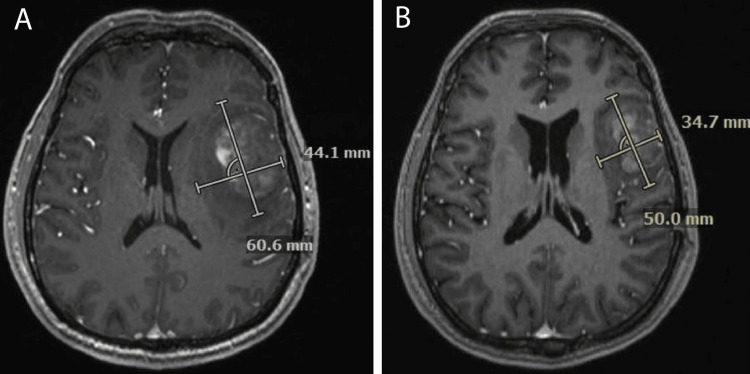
Radiographic response after three cycles of azacitidine and venetoclax Axial MRI brain with contrast. A. Tumor measurements before exposure to AZA/VEN. B. Decrease in the tumor volume of approximately 19.1% per RANO criteria. AZA/VEN: Azacitidine, venetoclax
